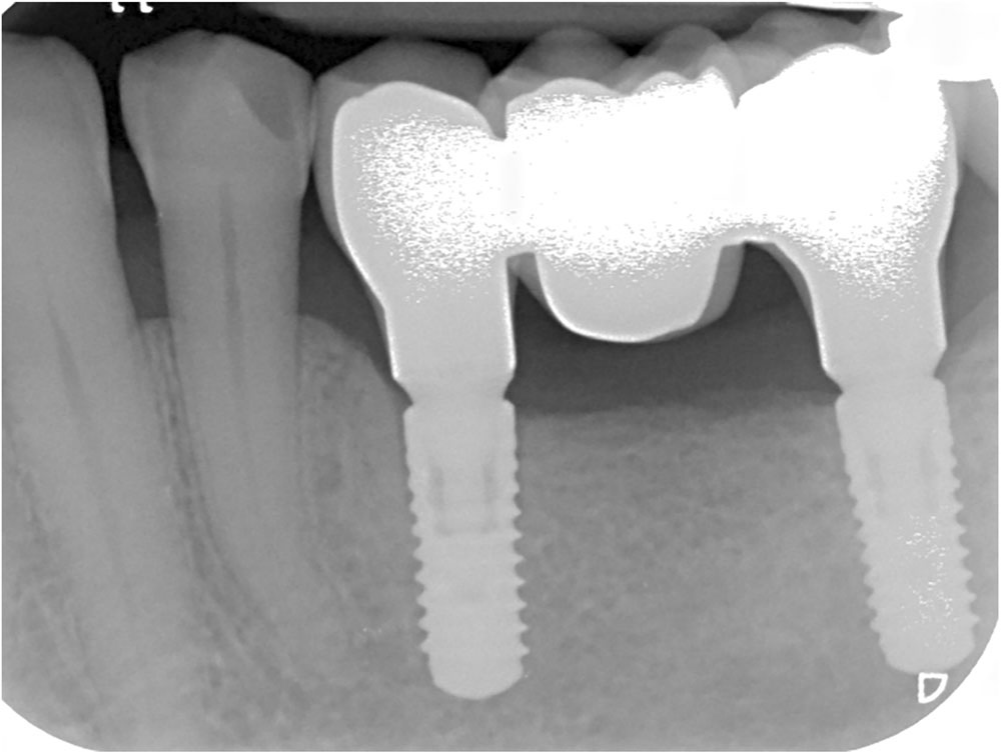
Sample radiograph of implant‐supported fixed dental prostheses, after a follow‐period of 2 years
